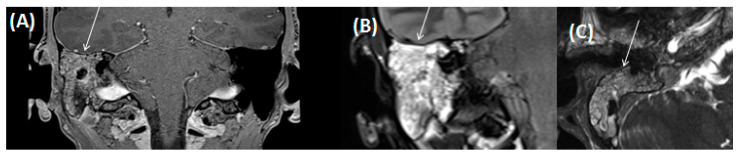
MRI of a 17-year-old male patient (same patient as shown in Figure 5 for CT) with coronal view for contrast-enhanced T1-weighted (A); coronal FLAIR (B); and axial CISS sequences (C). Note the complete obstruction of the mastoidal cells on the right side (arrow) as seen on the contrast-enhanced T1-weighted sequence (A) with discrete contrast enrichment of the mastoidal system. Total fluid obstruction is also demonstrated on FLAIR (B) and CISS sequences (C).
